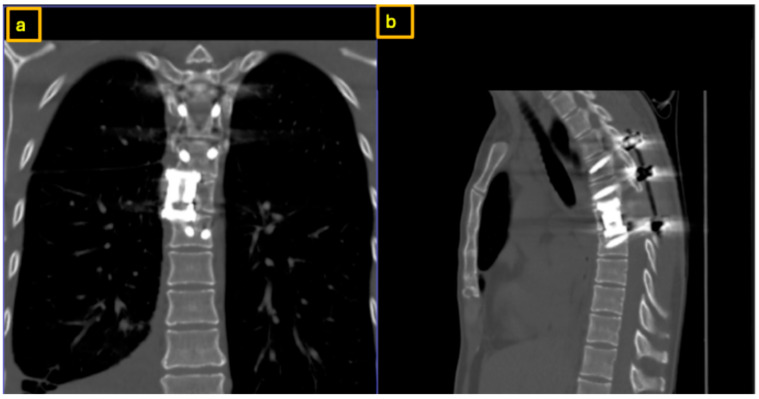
Postoperative CT-Scan at 3-month follow-up demonstrating hemi-vertebral body replacement at T7 right and posterior spinal fusion from T5 to T8: (a) coronal view, (b) sagittal view.
